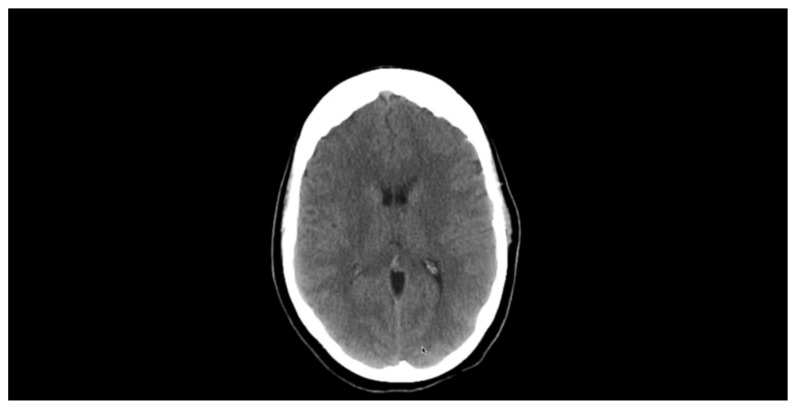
Brain CT showing complete resolution of scolexes following three-month albendazole therapy.
